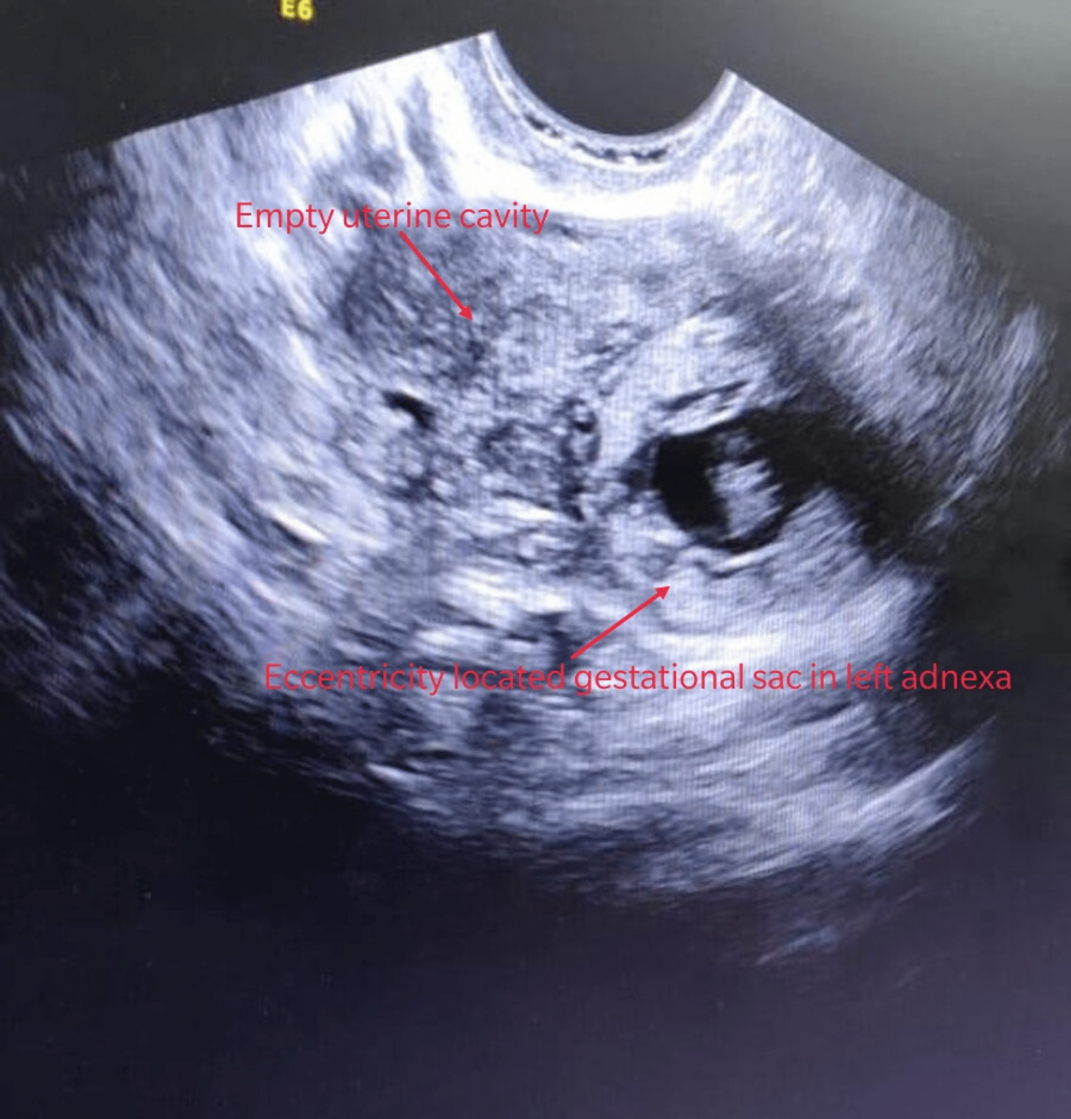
Ultrasound picture showing an empty uterine cavity and an eccentrically located gestational sac in the left adnexa
